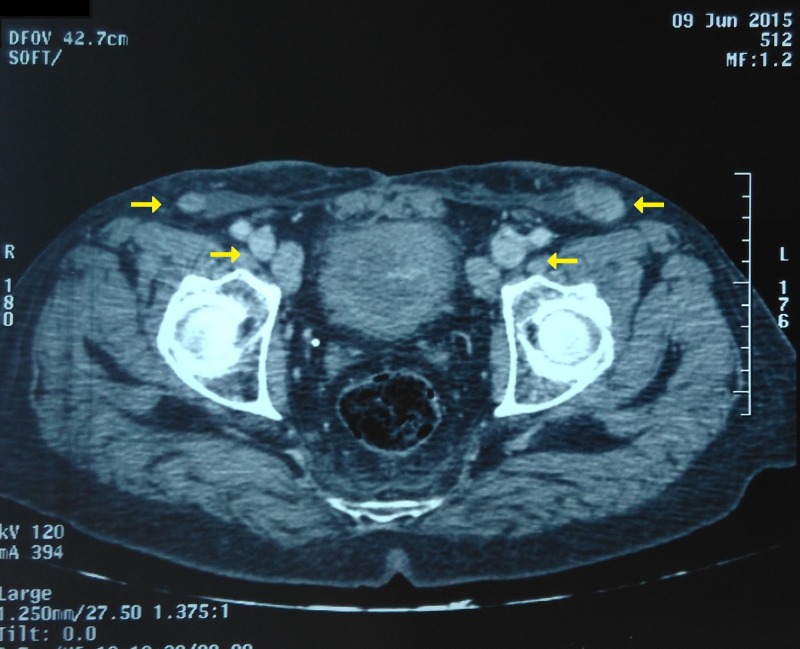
Axial section of CT scan showing bilateral iliac and inguinal lymph nodes (yellow arrows).
